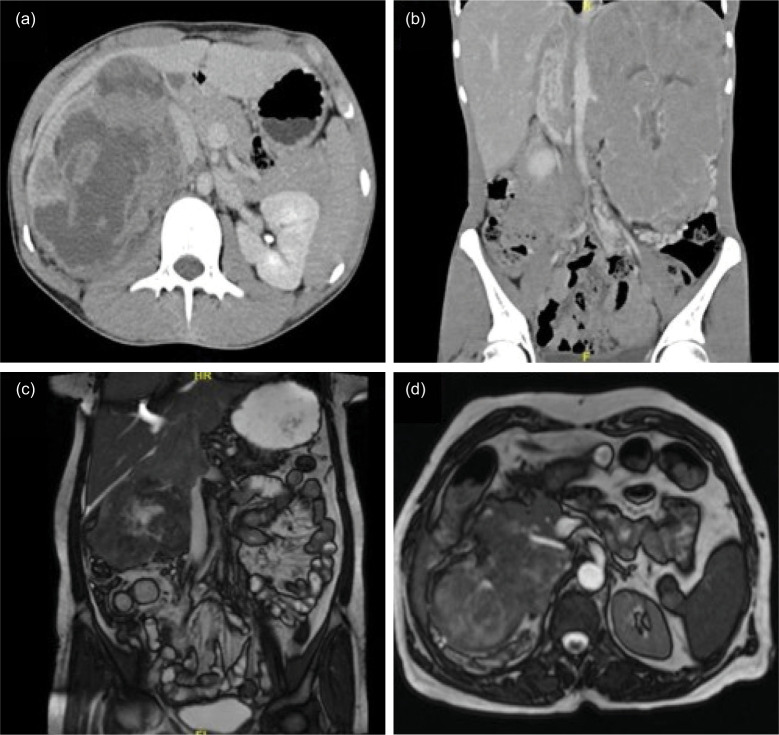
(a) CECT abdomen and pelvis transverse section (Case 1) showing right renal mass, compressing medially the right renal vein, ureter, and IVC with sectoral contact of about 180° with no apparent tumor thrombosis. (b) CECT thorax and coronal abdomen section (Case 2) revealed that left renal mass lesion with enhancing tumor thrombus extending up to the supradiaphragmatic IVC. (c) Plain MRI abdomen coronal section (Case 3) showing well-defined lobulated mass lesion with IVC thrombosis extending into the suprarenal IVC, below the diaphragm. (d) MR urography transverse section (Case 4) showing a well-defined mass of size 8 × 6 cm involving the renal pelvis, compressing IVC anteriorly. IVC, inferior vena cava; CECT, contrast-enhanced computed tomography.
